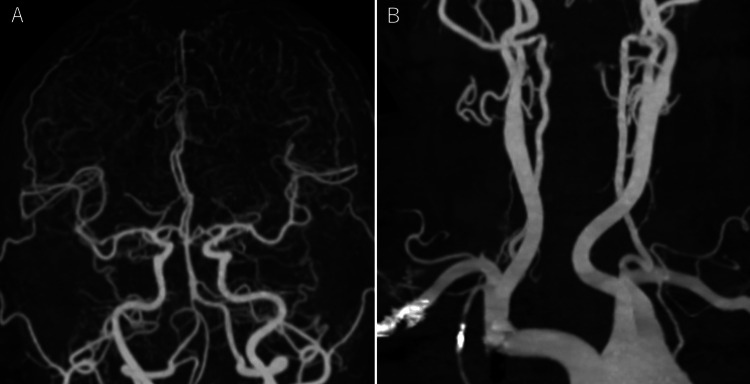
Three-dimensional computed tomography angiography showing no stenosis or dissection of the (A) cerebral or (B) carotid arteries.
